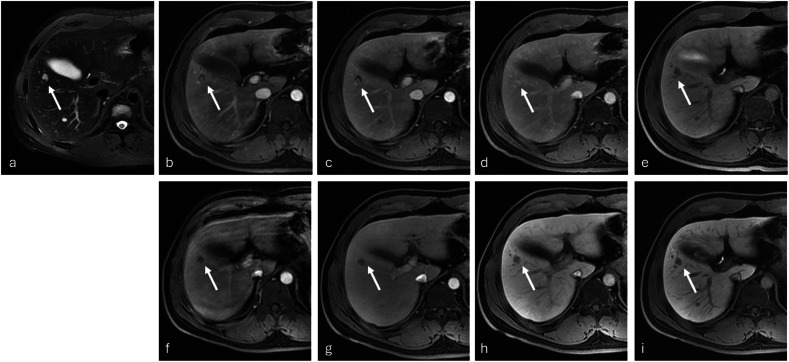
Small (1 cm) hemangioma. The unenhanced T2-weighted fat-saturated (a) image reveals a hyperintense lesion (arrow) in the right liver lobe, segment V. The lesion demonstrated enhancement features (“dot sign” enhancement and centripetal filling-in) characteristic of hemangioma on the late arterial (b), portal-venous (c) 5-min delayed (d) images after gadobenate administration followed by hypointensity in the HBP (e). Conversely, the lesion was hypointense during all corresponding dynamic phases (f–h, respectively) and the HBP (i) after gadoxetate administration leading to a diagnosis of metastasis. Note also the presence of motion artifacts on the early dynamic acquisition after gadoxetate administration. Imaging follow-up confirmed the diagnosis of a benign lesion, and imaging findings were consistent with hemangioma
